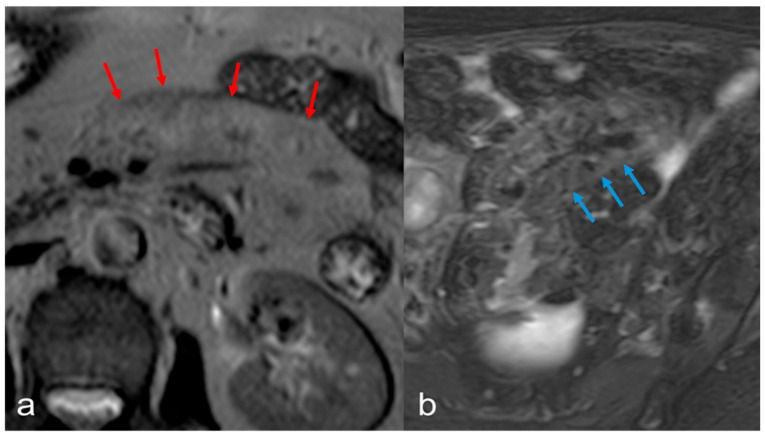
MRI findings in sigmoid diverticulitis: (a) axial MRI showing mesenteric panniculitis with a distinct pseudocapsule and mass effect (red arrows); (b) coronal MRI showing sigmoid diverticulitis with inflammatory bowel wall thickening (blue arrows).
